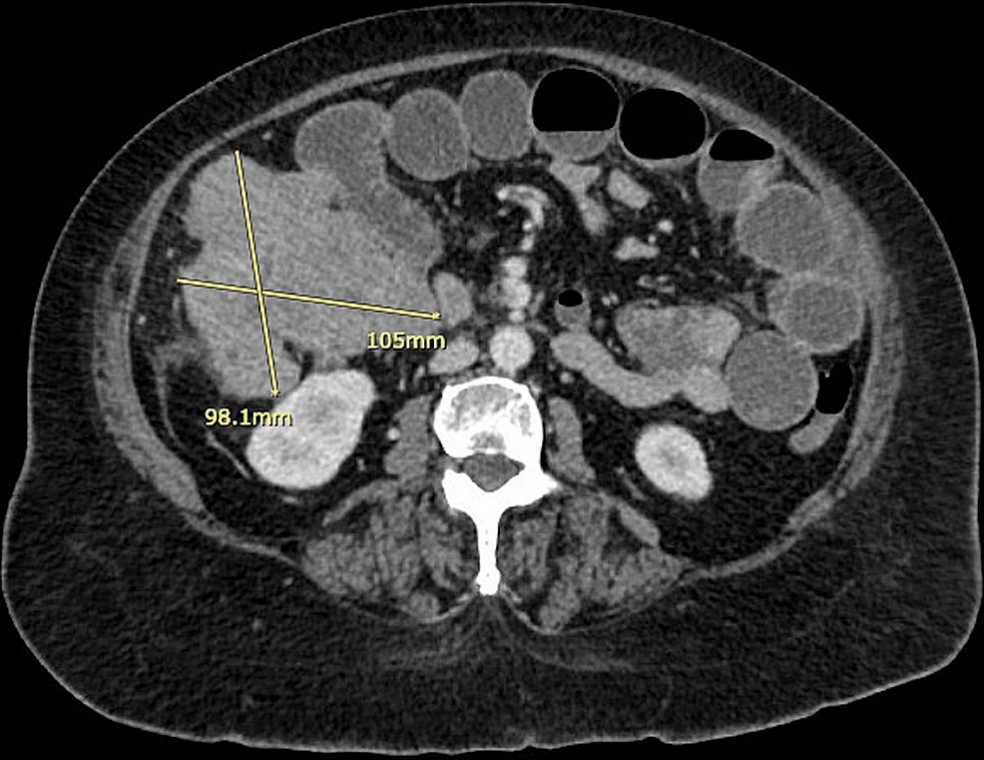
Computed tomography, cross-sectional view, showing the large right colon mass causing obstruction.
